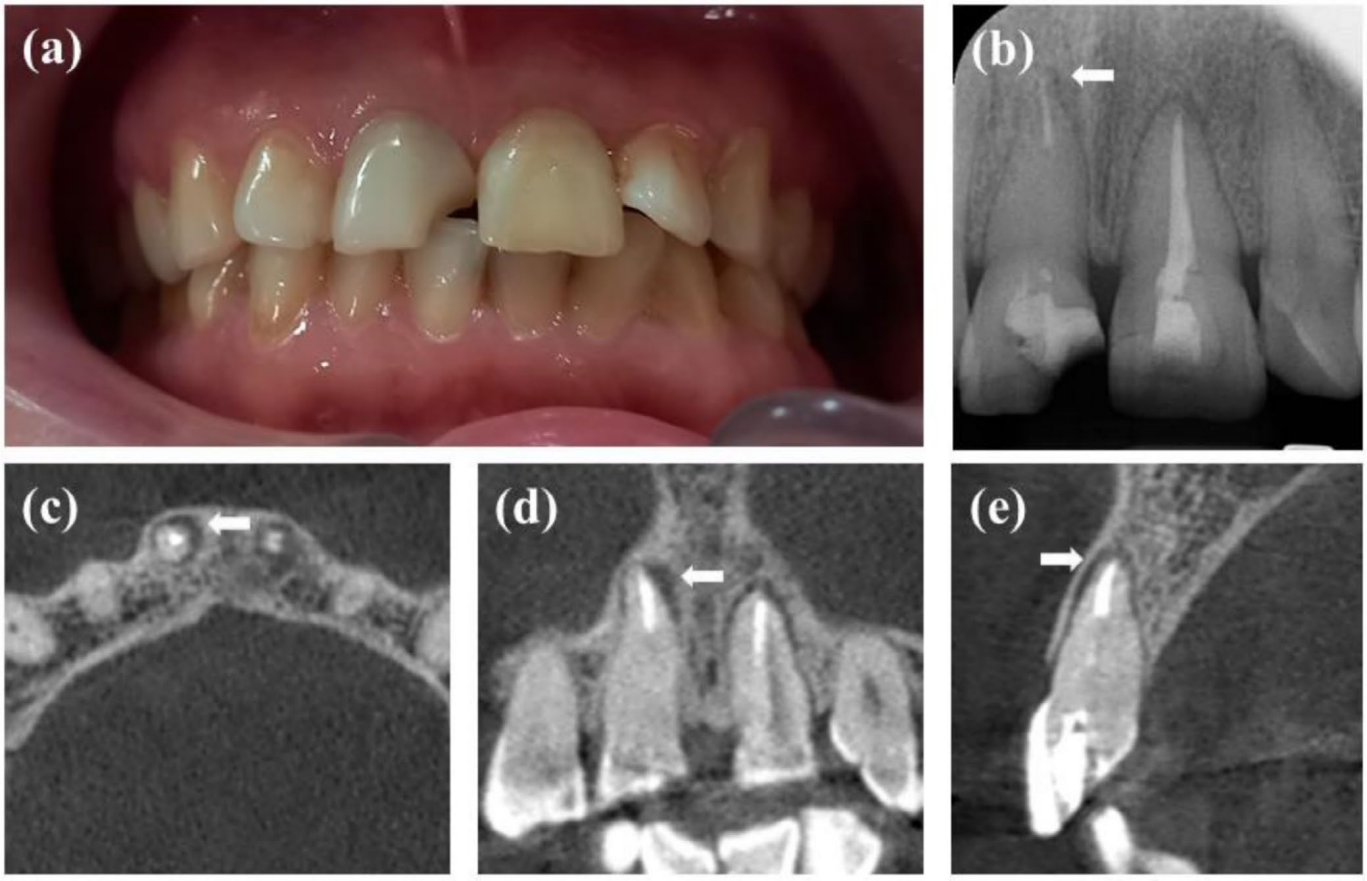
(a) Perioperative clinical photography showing a fractured tooth #8. (b) Preoperative radiography showing preinstalled fiber post and periapical lesion. (c–e) Preoperative CBCT assessment. Axial, coronal, and sagittal planes confirm a radiolucent area with intact buccal and palatal plates.
